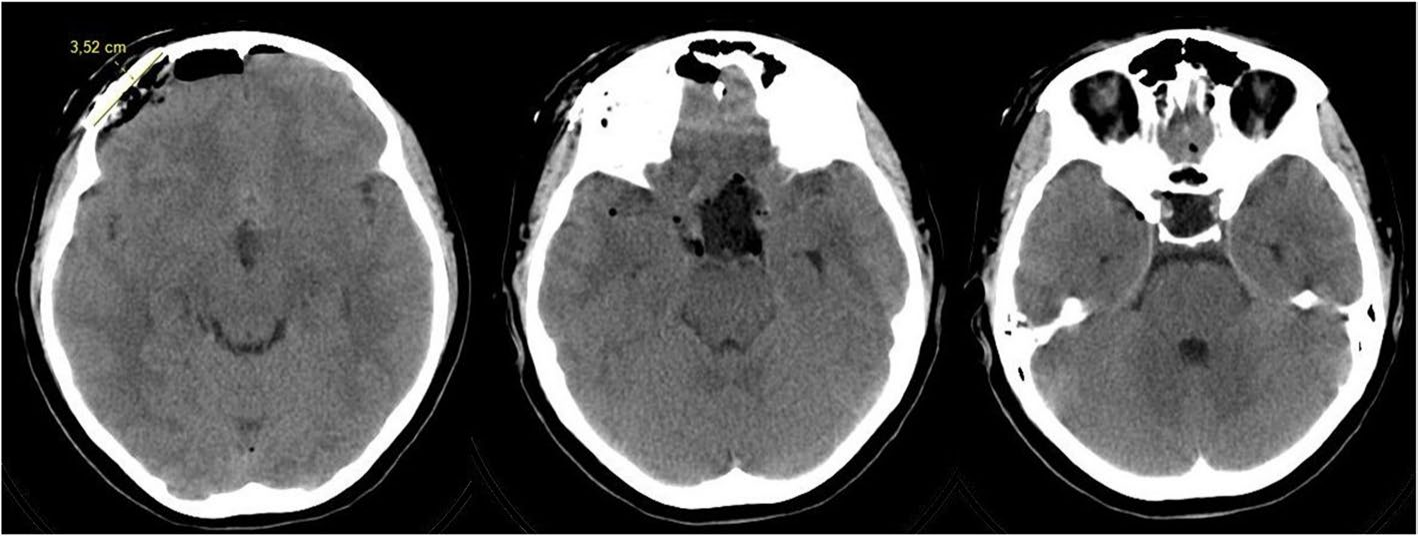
Postoperative (12 h after) CT scan, craniotomy size shown
